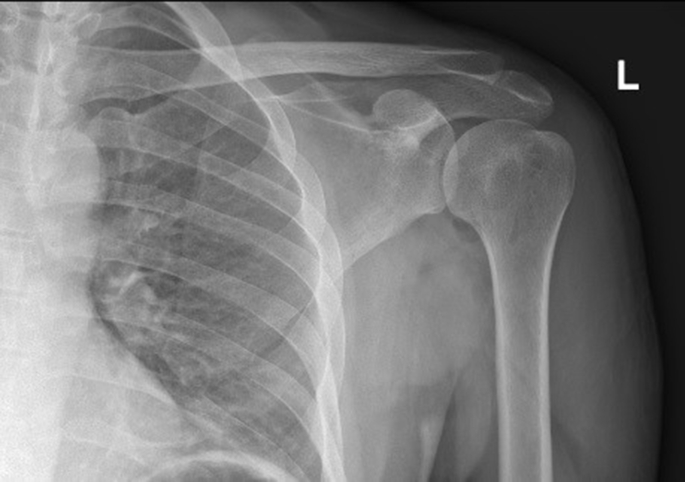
X-Ray showed post operative flat and complete removal of exostotic bone
